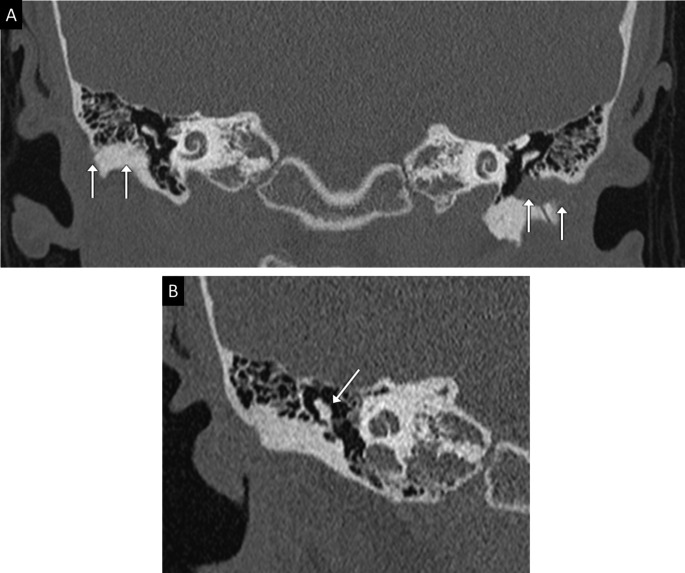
Computed tomography (coronal views) focused on the external and middle ear structures. (a) Bilateral complete aural atresia. It is fibro-osseous on the right side and fibrous on the left side (arrows). (b) Right malleolar dysplasia, the malleus is fused with the lateral wall of the tympanic cavity (arrows)
